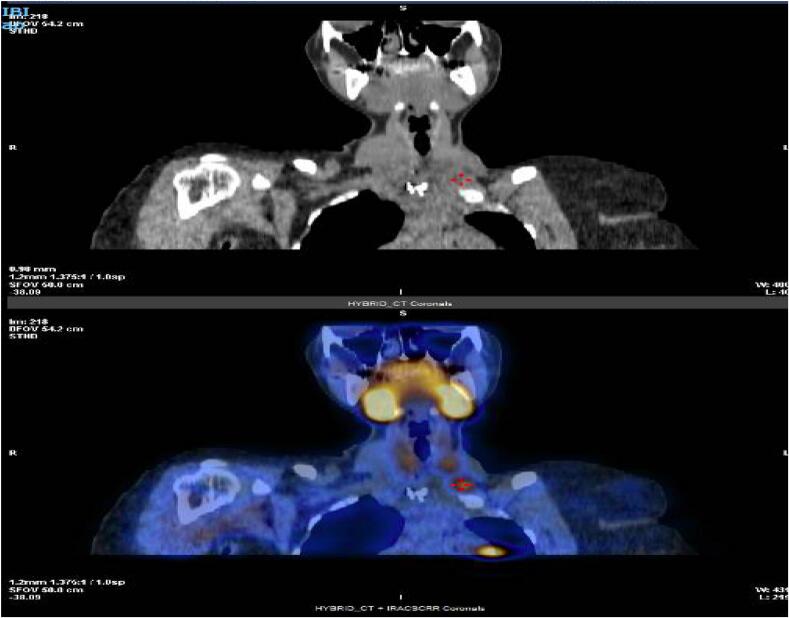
SPECT revealed hyperactivity of parathyroid tissue.
